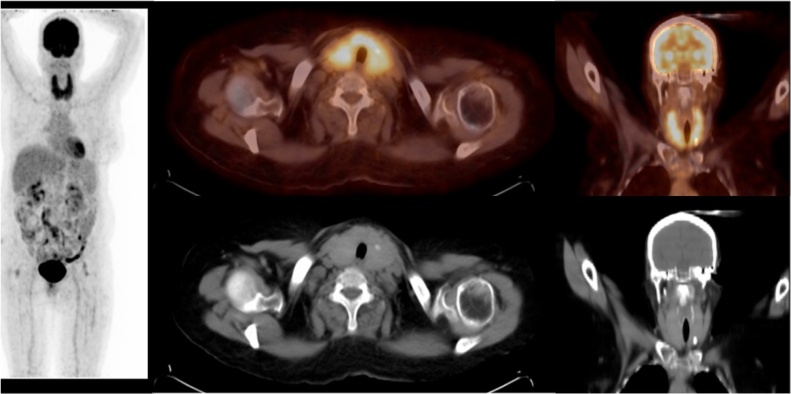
62 years old female with DM, HTN and cervical lymphadenopathy. F-18 FDG PET/CT showed diffusely enhanced FDG thyroid uptake, slightly heterogeneous CT morphology with diffusely reduced thyroid gland density, left lobe focal calcification, SUVmax of 4.6 and CT HU of 52. FNA cytological revealed autoimmune thyroiditis.
